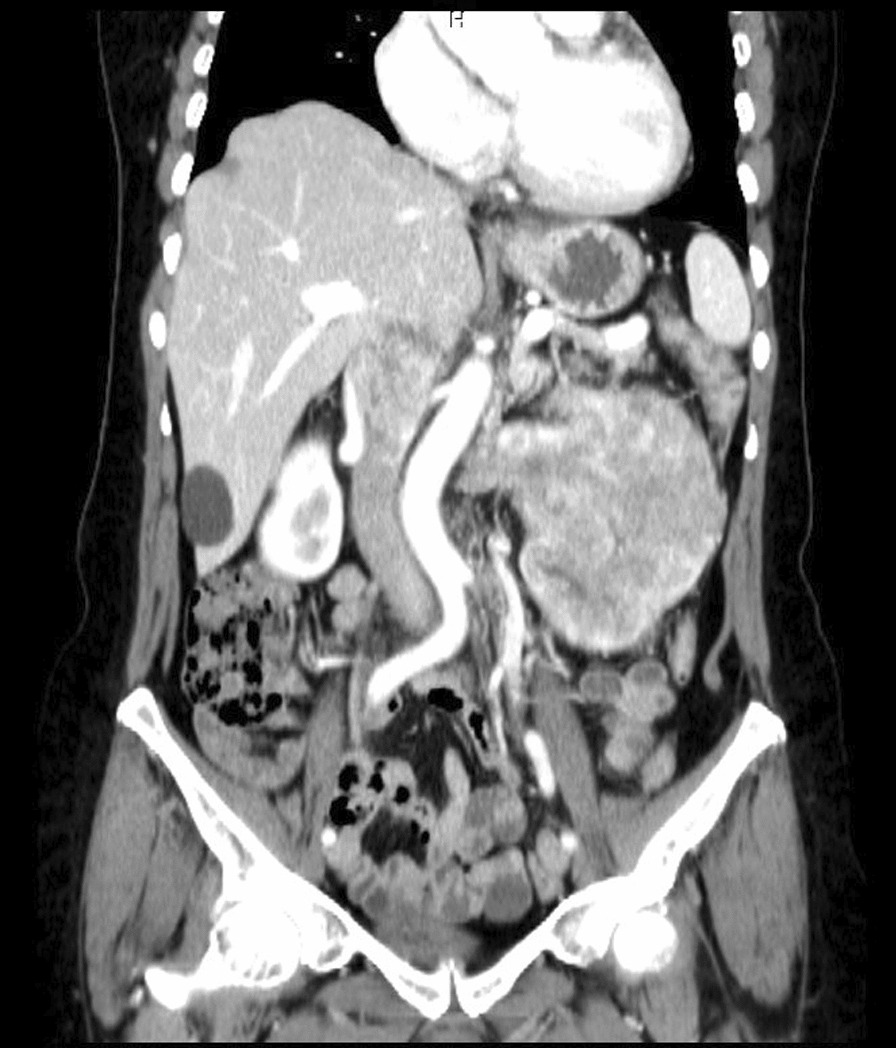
Preoperative computed tomography angiography, coronary view
